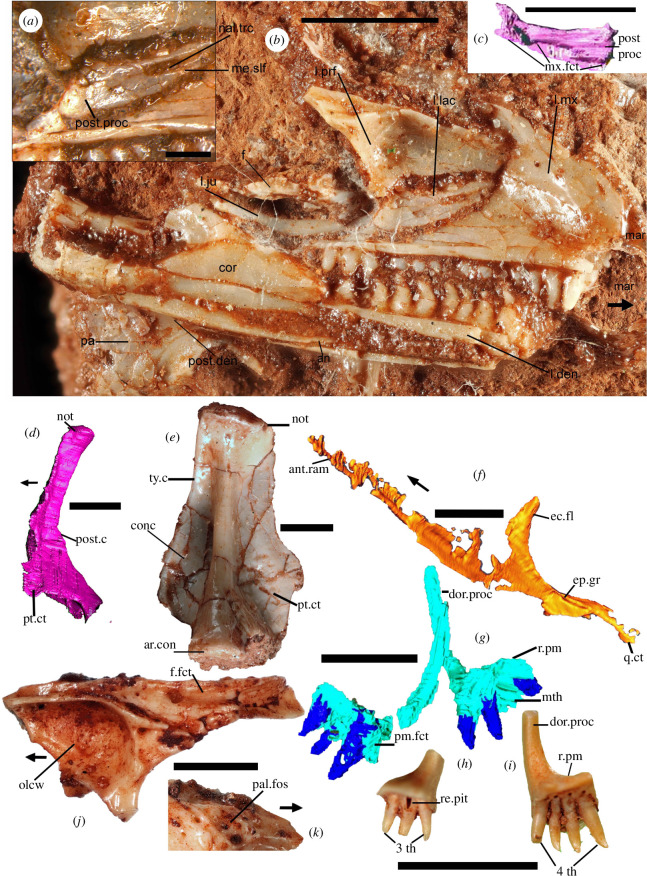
Photographs and CT scan images of NHMUK PV R36822 holotype bones of *†Cryptovaranoides microlanius* and isolated quadrate, prefrontal and premaxillae. (*a–c*) Left side of holotype skull and lower jaw of *†Cryptovaranoides microlanius*. (*a*) Close-up of left lacrimal in medial view. (*b*) Left side of skull and lower jaw in medial view. (*c*) Computed tomography (CT) scan of left lacrimal in lateral view. (*d*) Holotype right quadrate CT scan in medial view. (*e*) Isolated left quadrate NHMUK PV R 37606 digitally removed from matrix. (*f*) CT scan image of holotype right pterygoid in ventromedial view. (*g*) CT scan of holotype left and right premaxillae in posteroventral views. (*h*) Isolated left premaxilla NHMUK PV R 38914 in posteroventral view. (*i*) Isolated right premaxilla NHMUK PV R 38913 in posterior view. (*j*) Isolated right prefrontal NHMUK PV R 38912 in medial view. (*k*) Lateral view of right prefrontal NHMUK PV R 38912 showing palpebral fossa. Scale bars all 2 mm except (*a*) which is 0.5 mm. Arrows show anterior. an, angular; ant, anterior; ar, articular; c, crest; con, condyle; conc, conch; cor, coronoid; ct, contact; den, dentary; dor, dorsal; ec, ectopterygoid; ep, epipterygoid; f, frontal; fct, facet; fo, foramen (foramina); fos, fossa; gr, groove; ju, jugal; l., left; lac, lacrimal; mar, missing anterior region; me, medial; mth, missing tooth; mx, maxilla (maxillary); nal, nasolacrimal; not, notch; olcw, olfactory chamber wall; pa, palatine; pal, palbebral; pm, premaxilla; post, posterior; proc, process; prf, prefrontal; pt, pterygoid; ram, ramus; re, resorption; q, quadrate; r, right; slf, shelf, th, tooth (teeth); trc, tract; ty, tympanic.
